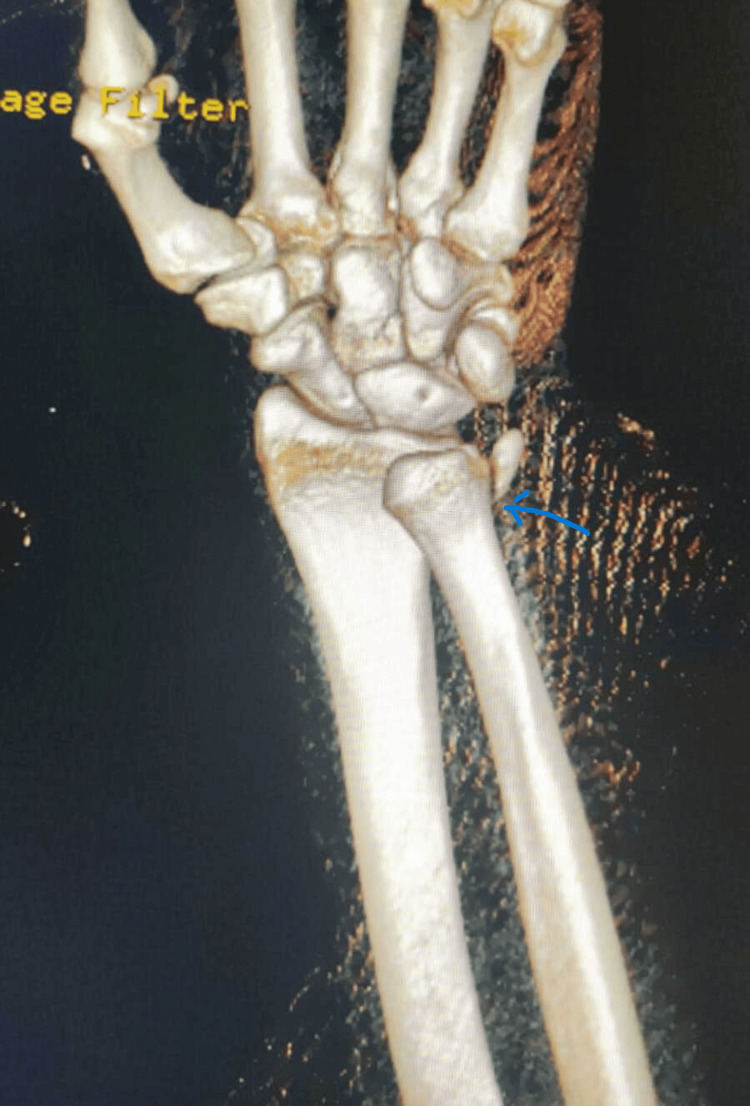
3D computed tomography Computed tomography 3D reconstruction clearly showing volar distal radioulnar joint dislocation associated with an ulnar styloid avulsion fracture (from another view). Blue arrow pointing to the ulnar styloid avulsion fracture with the head going to the volar surface of the radius. Figure created using Philips IntelliSpace PACS (formerly known as iSite) (Amsterdam, Netherlands).
